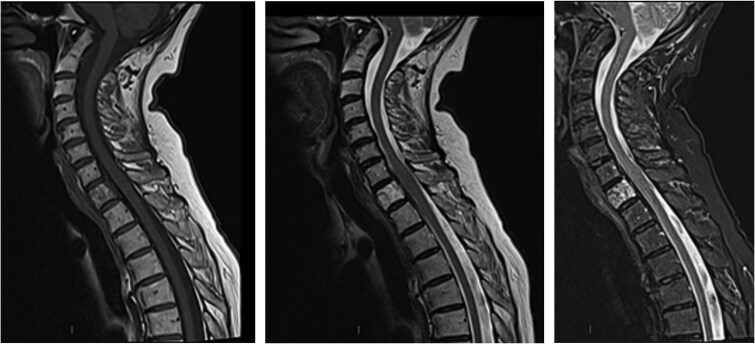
T1W, T2W, STIR sagittal MRI imaging demonstrating a vertebral haemangioma at the level of the first thoracic vertebra. No loss of vertebral body height or alignment.
